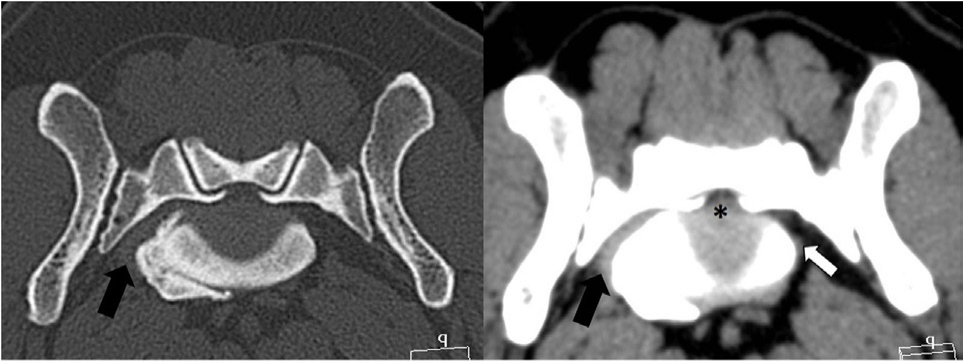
Transverse CT images of the bone and soft tissue window from the patient’s lumbosacral foramen showing a moderate disk protrusion (*) and a ventrolateral spondylosis, resulting in stenosis of the right foramen. The left L7 nerve is of normal size (white arrow); however, the right L7 nerve cannot be clearly identified within the soft tissue proliferation (black arrow).
